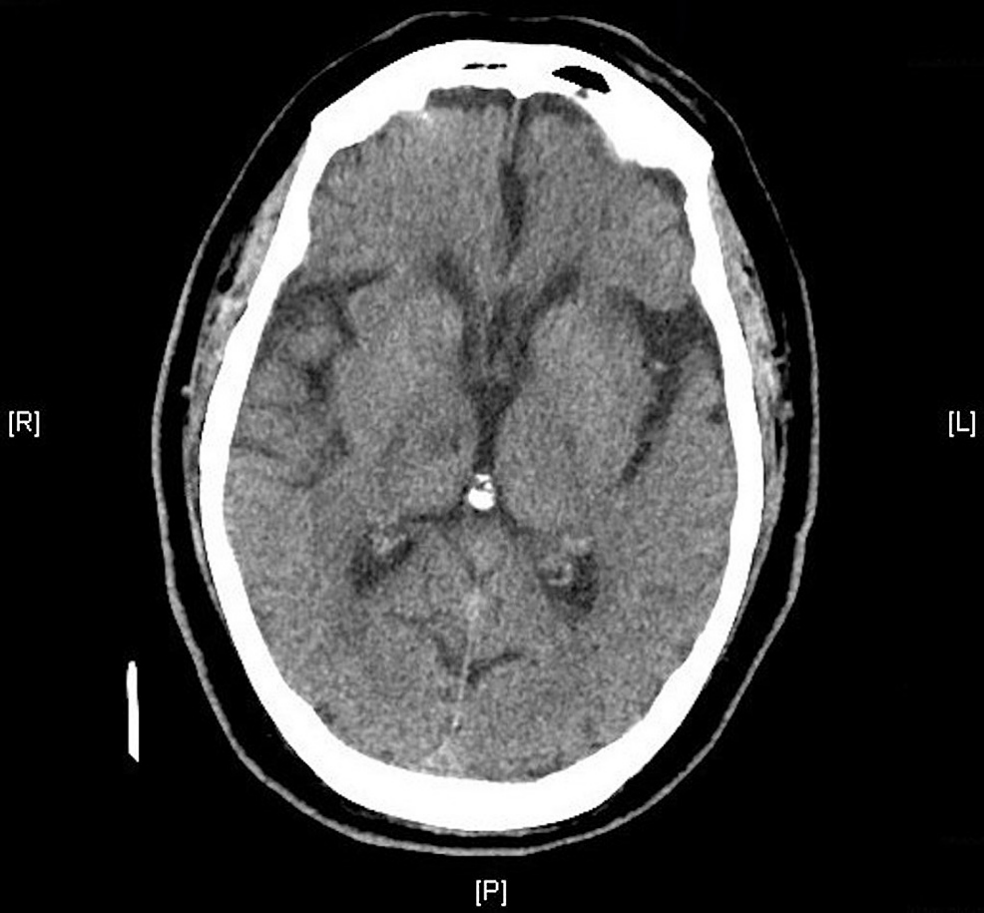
Initial “stroke alert” CT, which did not reveal any evidence of hemorrhage or infarct. CT, computed tomography
